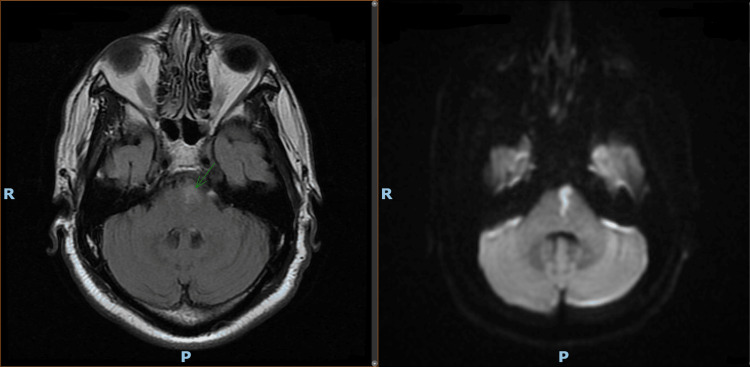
Axial T2 FLAIR MRI (left) and diffusion-weighted imaging (right) There is a small area of acute/subacute infarct in the left paramedian pons seen on T2 FLAIR MRI (green arrow). Diffusion-weighted imaging shows the same infarct.
